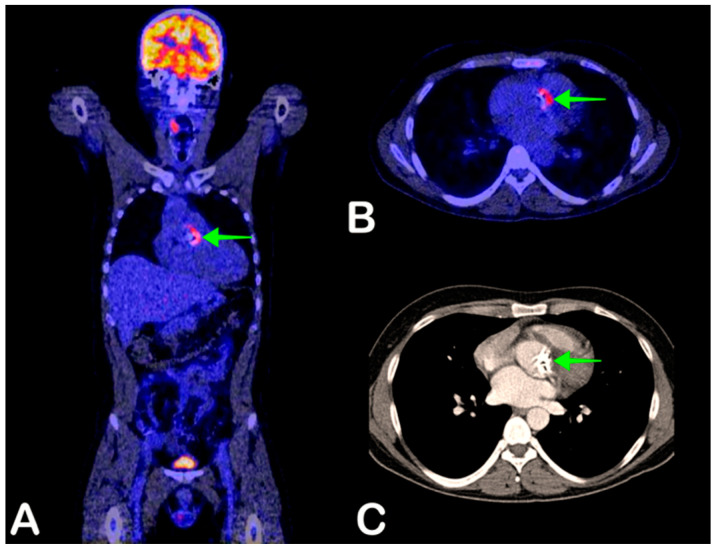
A 39-year-old man presented with general malaise for one week, fever of 41 degrees Celsius, and petechiae. Blood cultures were positive for *Streptococcus pneumoniae*. One year earlier, his native aortic valve was replaced with a mechanical valve. Endocarditis was strongly suspected, but transthoracic and transesophageal ultrasound did not prove valvular vegetations or other signs of infection. Fused coronal and axial FDG-PET/CT showed FDG avidity of the prosthetic aortic valve suggestive of infection (A and B, green arrow). No other infection foci were found. The aortic valve is also shown on full-dose thoracic CT (C, green arrow), without any anatomical signs of infection. The aortic valve was surgically replaced after which the patient recovered.
